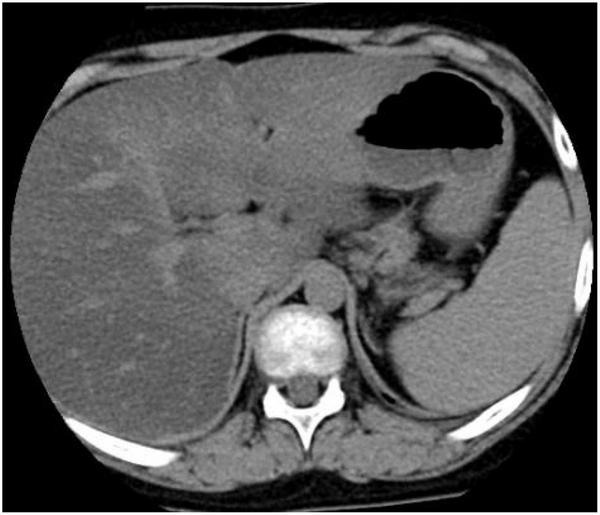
Computed tomography (CT) scan of the adrenal gland showed nodular thickening of the left adrenal junction of patient 4.
